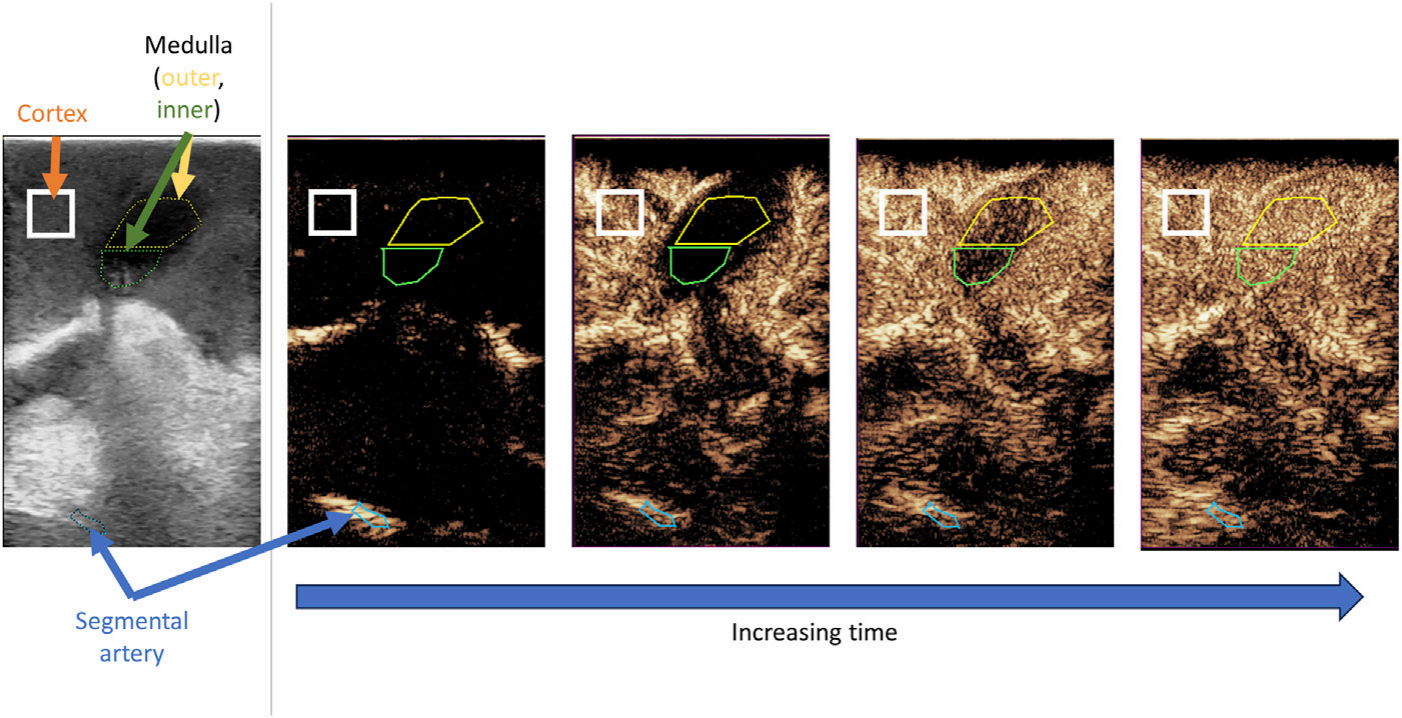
Representative contrast enhanced ultrasound loop with regions of interest selected. Standard B mode ultrasound image (left panel) is used to ensure cortex and medulla are in view. As time progresses there is sequential perfusion of segmental artery, cortex, outer medulla and inner medulla.
